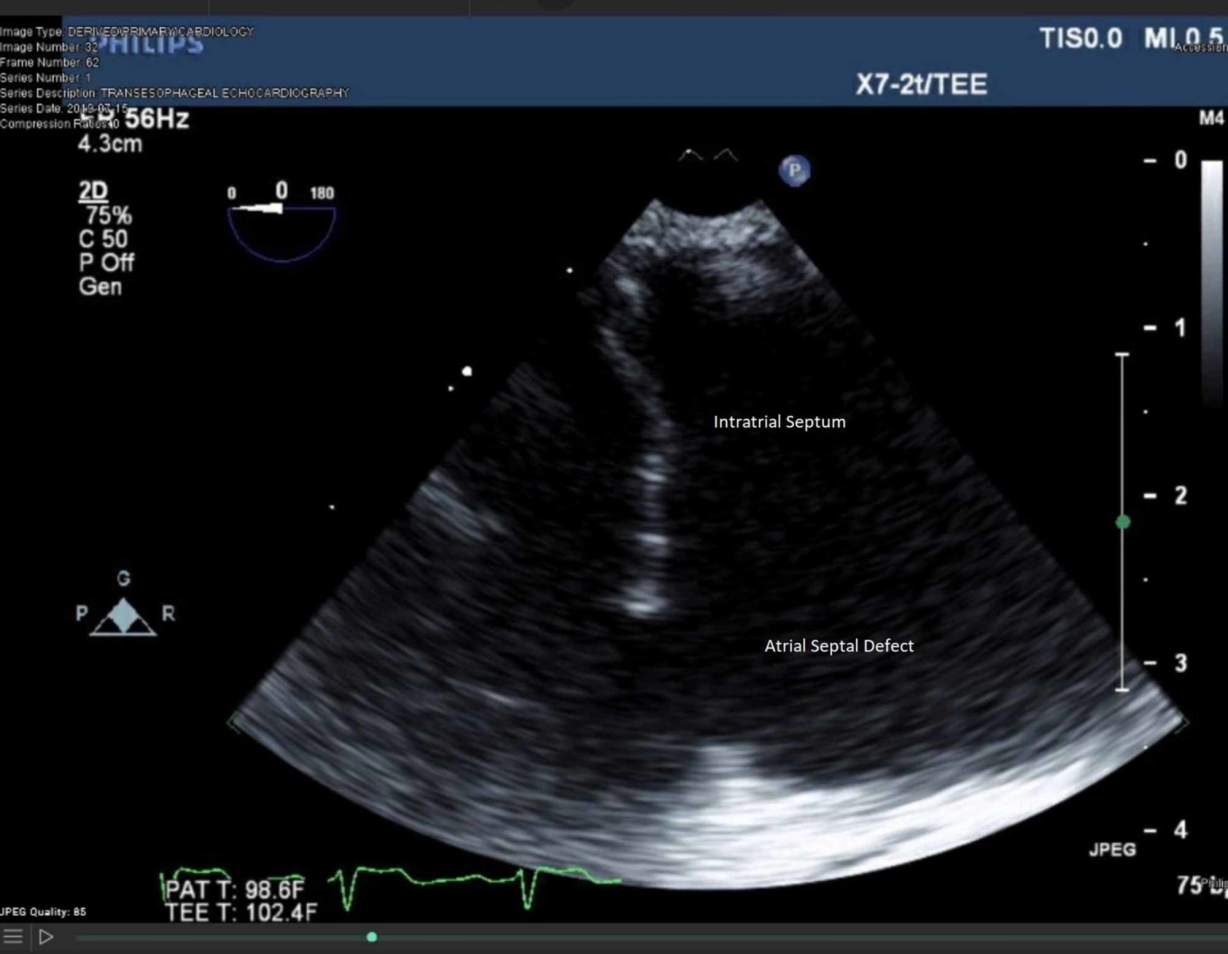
Transesophageal echocardiogram showing an atrial septal defect
